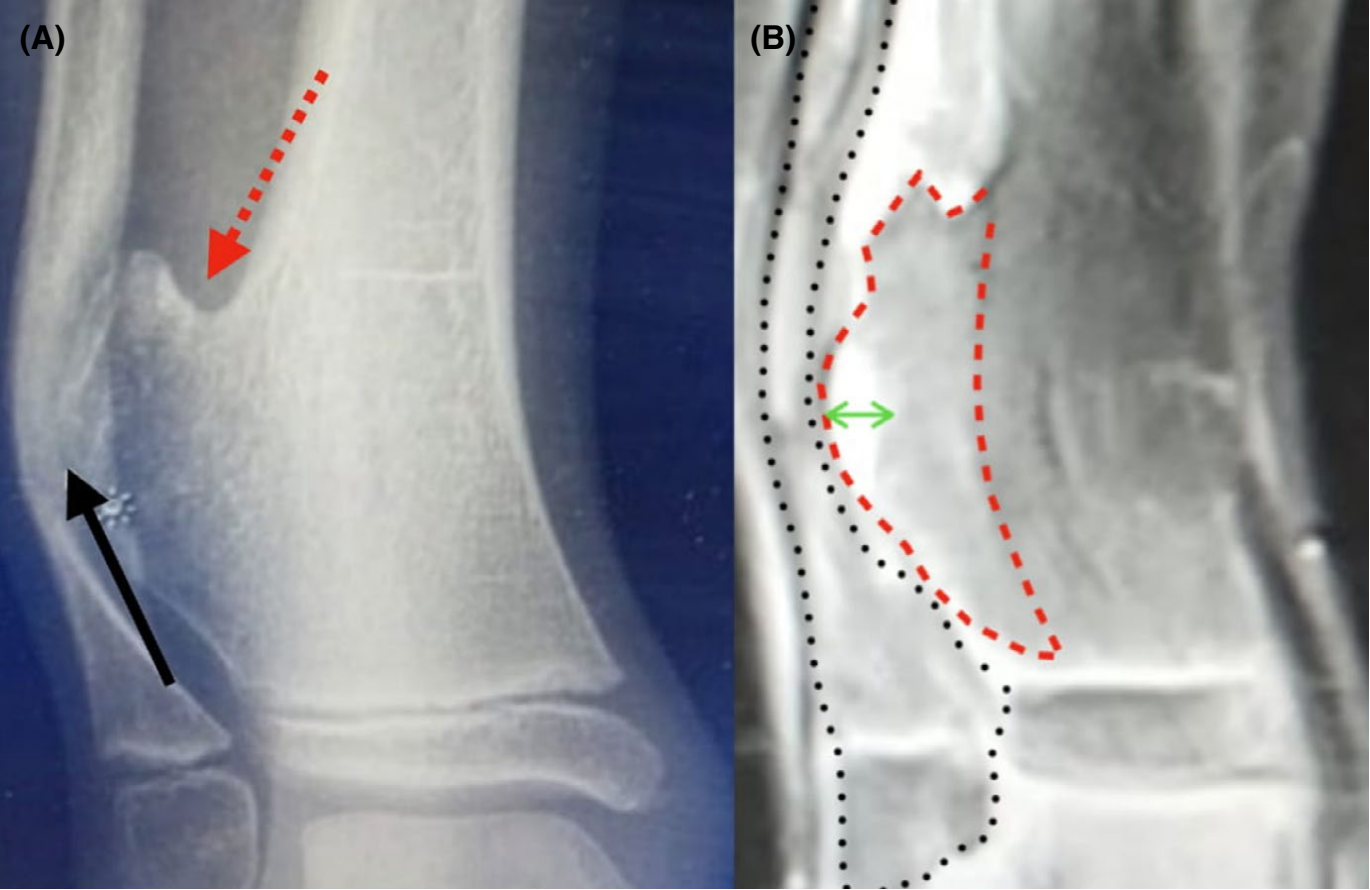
A, AP view of the distal tibia. The red dotted arrow shows the osteochondroma of our patient. The black arrow shows a significant bowing and weakening of the fibula. B, MRI view of the distal tibia of our patient. The red dotted circle shows the osteochondroma that expands and “pressures” the fibula. The green arrow shows the cartilage cap, which should always be measured. The black dots indicate the bowing of the fibula
